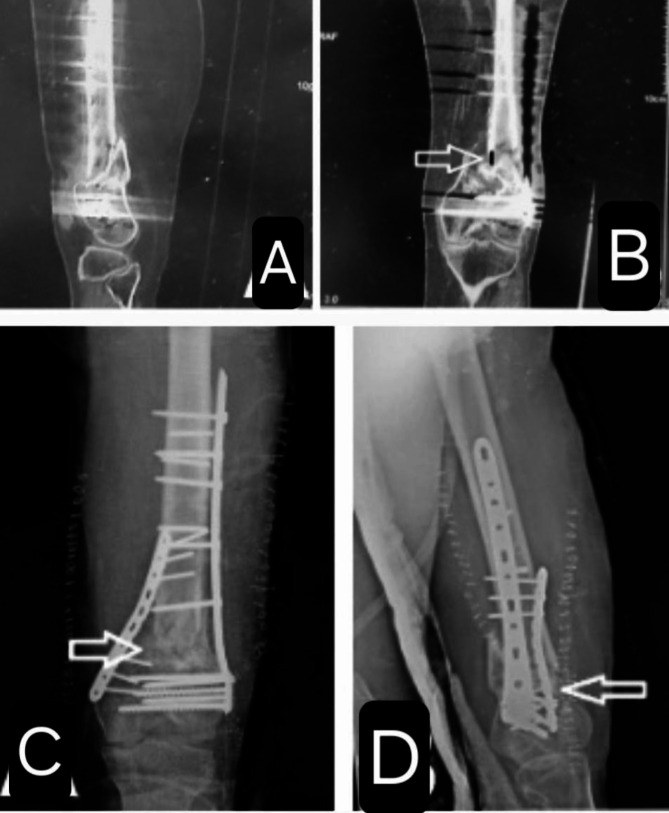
Preoperative and postoperative radiographs showing fracture nonunion and subsequent surgical intervention. Images A and B are preoperative views, with A showing the lateral view and B the anteroposterior (AP) view; in B, the white arrow indicates the nonunion gap, and the black line demonstrates a length of approximately 10 mm, as measured by the radiographic scale. Images C and D are postoperative X-rays, with C showing the AP view and D the lateral view; in both views, the white arrow indicates the medial femoral condyle corticoperiosteal flap applied to the anteromedial aspect of the femur
